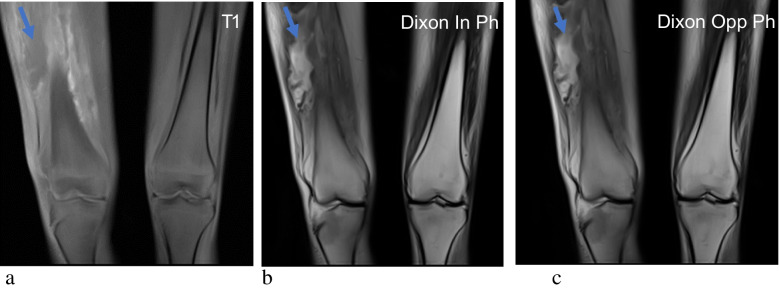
SABM in a 35-year-old female with a background of diabetes mellitus and myonecrosis. T1-weighted image (a) demonstrates the characteristic intermediate to mildly hypointense marrow signal in SABM. No visible difference in bone marrow signal between the coronal T2 Dixon in-phase (b) and opposed-phase images (c), resulting in nearly identical images and minimal chemical shift. An intramuscular haematoma was also demonstrated in the vastus lateralis (blue arrows)
